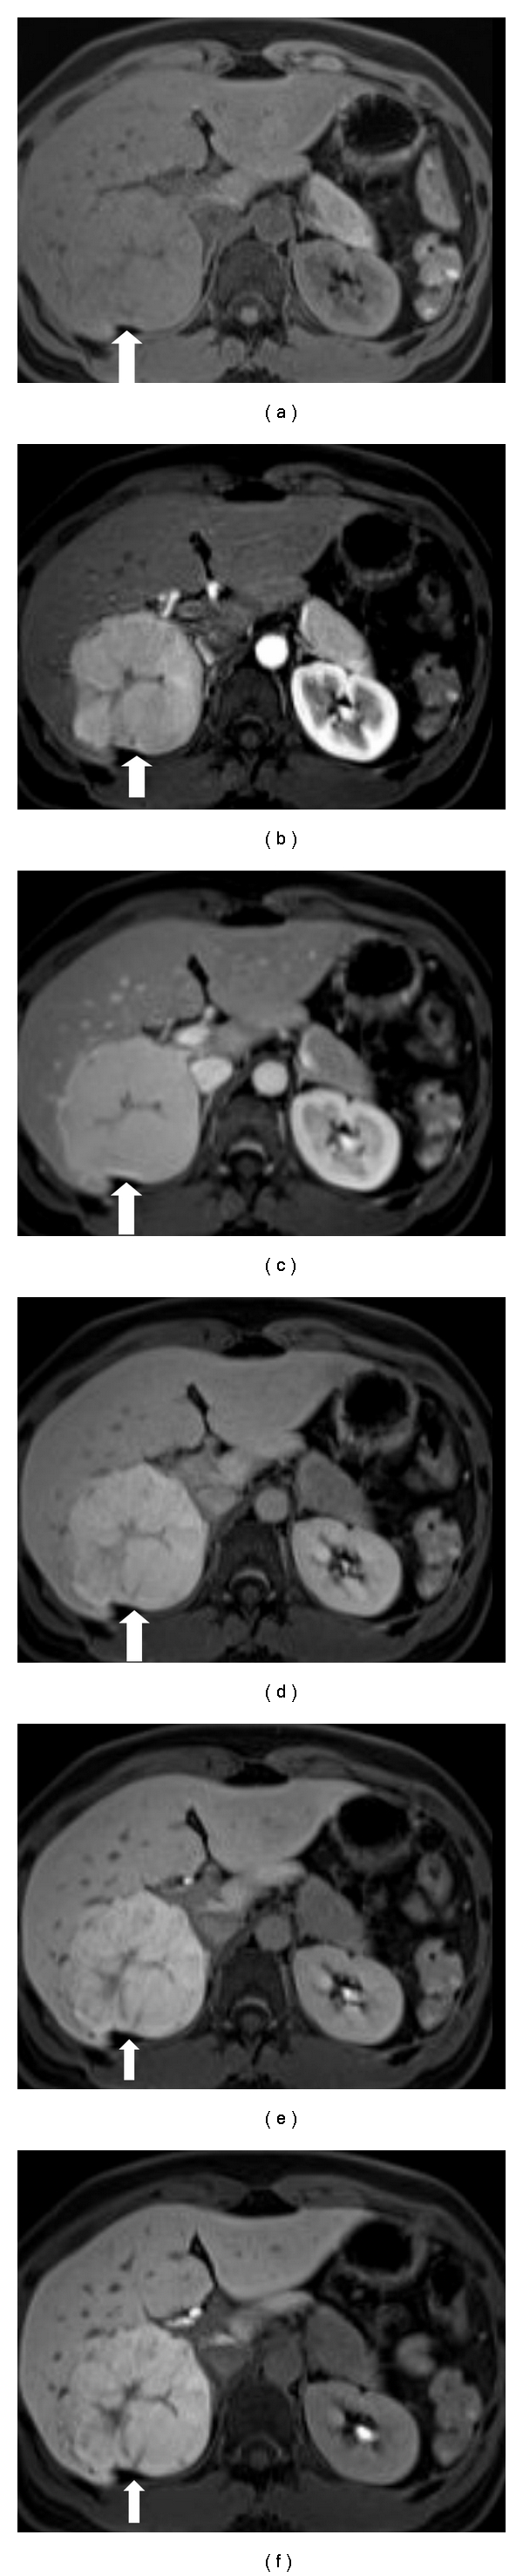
(a–d) Gadoxetate acid-enhanced MRI in the precontrast, arterial, venous, and equilibrium phases of 37-year-old female non-Hepatitis B or C virus carrier showing a large mass in the right lobe of the liver with early arterial enhancement and persistent late-phase enhancement with a small central hypointense scar. (e, f) Gadoxetate acid-enhanced MRI in the hepatobiliary phase 10–20 minutes after injection showing progressively hyperintense enhancement, in a homogeneous pattern apart from the hypointense small central scar. The MRI findings are typical for focal nodular hyperplasia.
